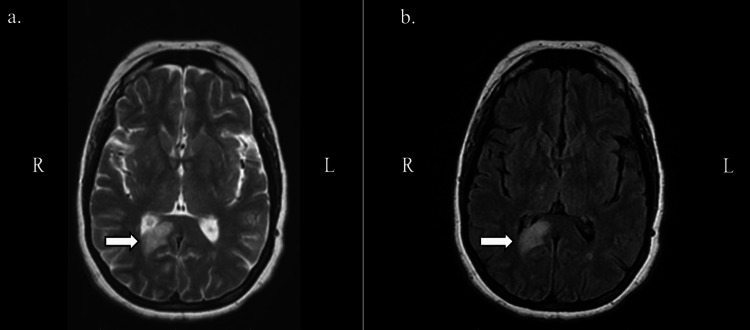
MR brain with and without contrast: inferior view. Transverse inferior view of MR brain: (a) fast spin echo and (b) FLAIR showing increased T2/FLAIR signal in the periventricular and subcortical white matter. MR: magnetic resonance, FLAIR: fluid-attenuated inversion recovery.
